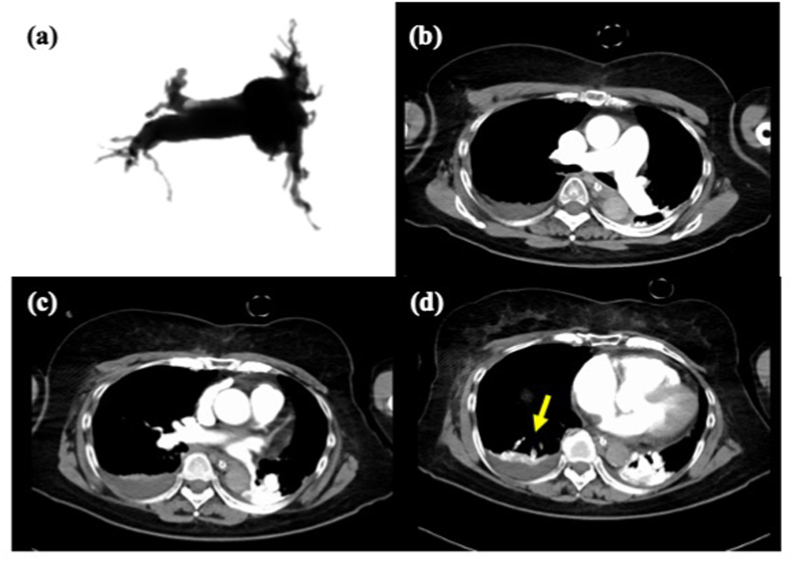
The computed tomography (CT) pulmonary angiography showed a small fresh thrombus in the right A10 (yellow arrow) and chronic thromboembolic obstruction of the bilateral distal pulmonary artery. (For interpretation of the references to colour in this figure legend, the reader is referred to the Web version of this article.)
